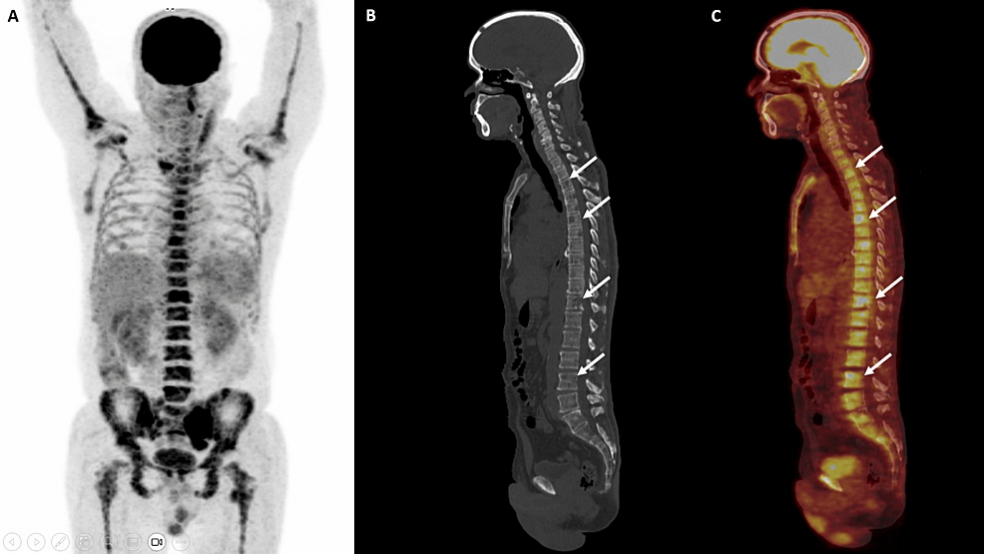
Maximum intensity projection image (A) reveals foci of increased FDG uptake at multiple axial and appendicular skeletal sites. Sagittal CT image (B) and fused PET CT image (C) reveal multiple FDG avid lytic lesions involving the spine (white arrows).
